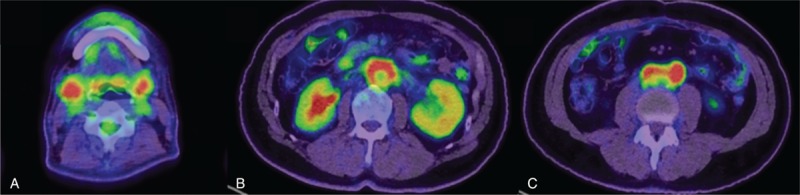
Positron-emission computed tomography findings. The abnormal accumulation was shown in submandibular glands (A), kidney (B), and periaorta (B, C).
